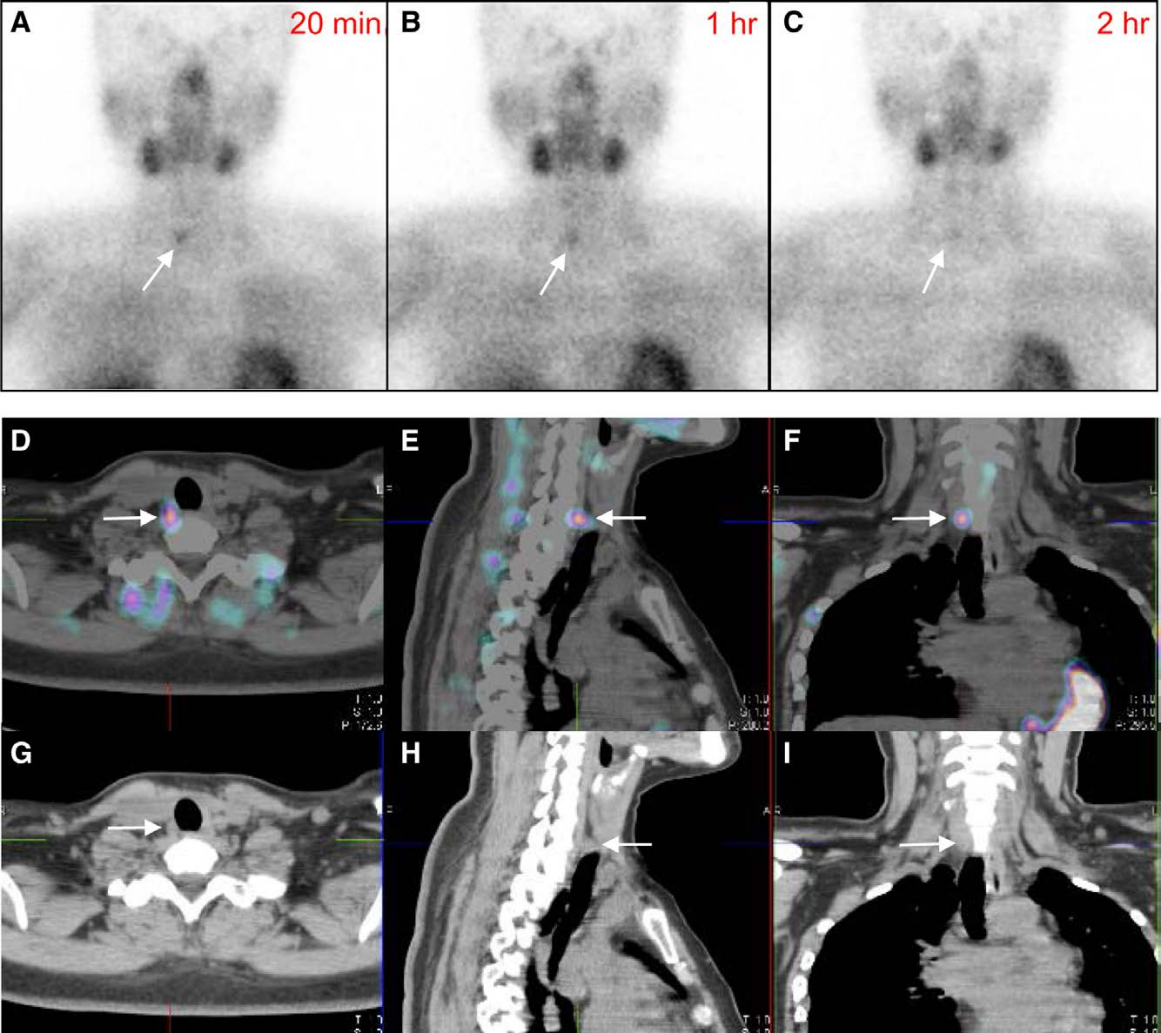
The second Tc-99m MIBI scan and SPECT/CT. Mild focal uptake was observed in the right lower thyroid area in the 20-min image (A). This uptake was sustained on the 1-h and 2-h images (B, C). Additional SPECT/CT revealed a 0.8 × 0.4-cm sized nodular lesion in the lower portion of the right thyroid bed (D–F, fusion images, G–I, CT images). Arrows indicate the lesion.
